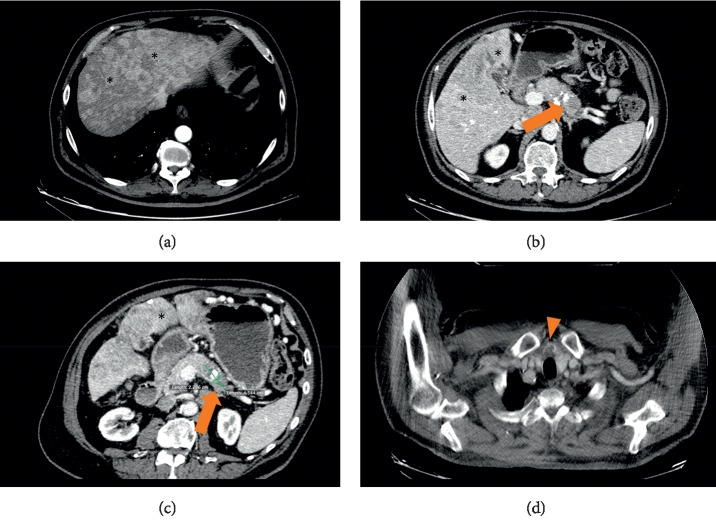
CT images (a)–(c) show multiple hypervascular liver metastases (*∗*) and a focal solid lesion of the pancreatic tail (arrow). A thyroid nodule (arrow head) was also detected (d).
